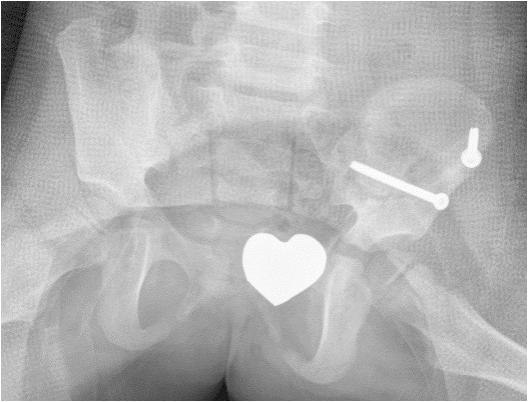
The postoperative pelvic X-ray in a spica cast shows anatomic reduction of the left acetabular fracture.
